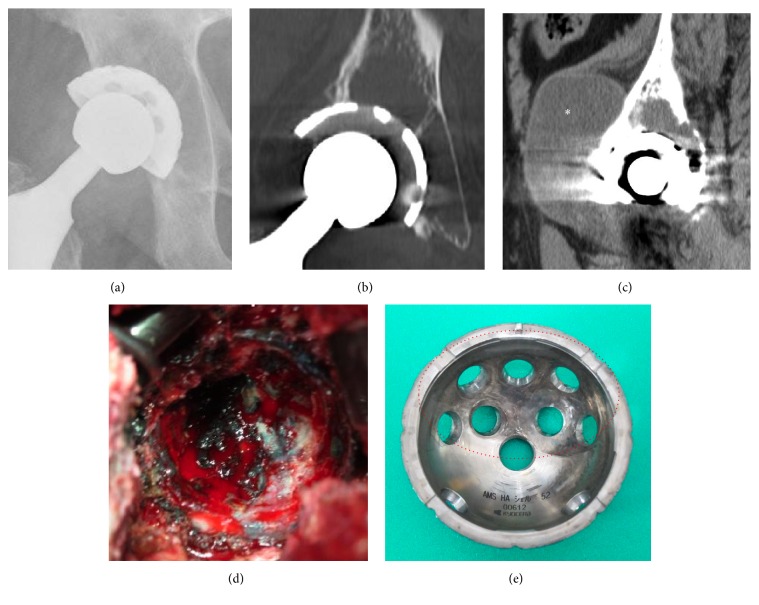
An anteroposterior view of plain radiograph (a) and a CT coronal image (b) showing the expanding retroacetabular osteolysis. A pseudotumor at the anterior aspect of the right hip (asterisk) connected to the hip joint was observed in a CT sagittal image (c). Pigmentation of metallic debris inside the osteolytic lesion and around the joint capsule and acetabulum was seen intraoperatively (d). A photograph of the retrieved cup (e) showing abrasion inside the metallic shell connecting to polyethylene liner (dotted circle).
